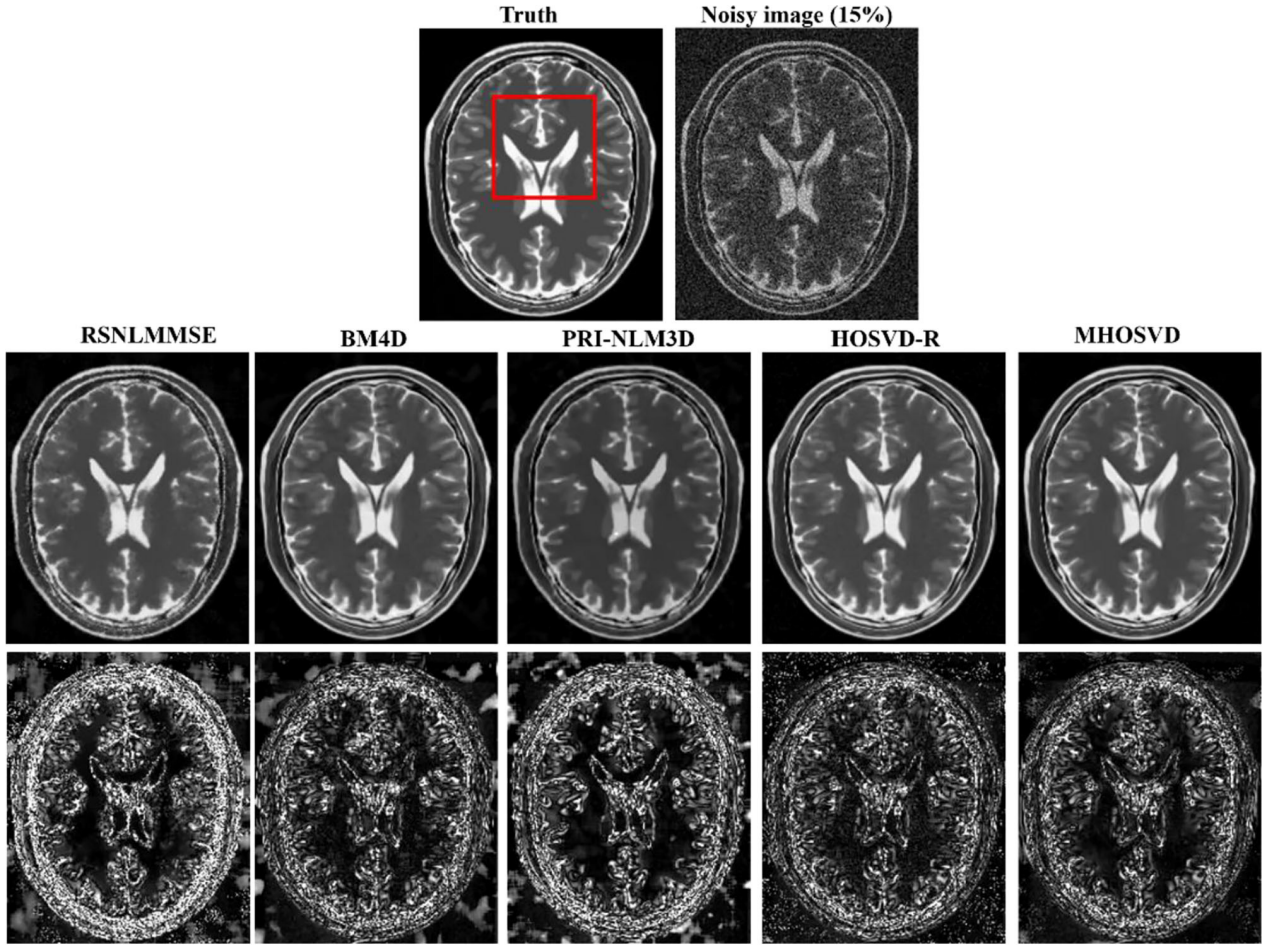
Denoising T2-weighted (T2w) images by different methods under 15% Rician noise. First line: T2w image without noise and with 15% Rician noise. Second line: the denoised images with four different methods. Third line: the corresponding error images.
